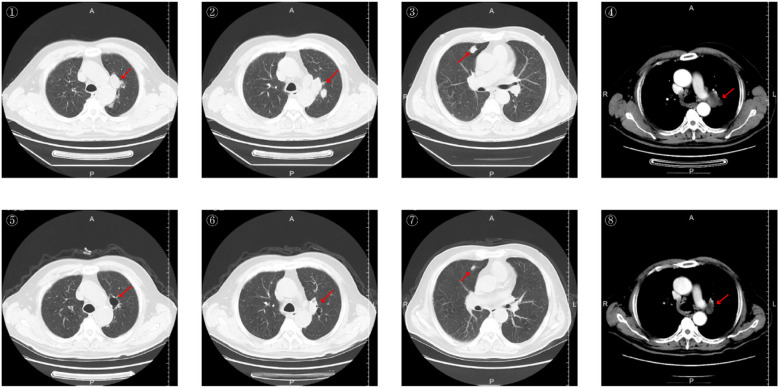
Chest CT images before and after treatment. Images ①②③④ show pre-treatment chest CT scans taken on July 17, 2025, revealing a mass at the hilum and within the left lung. Images ⑤⑥⑦⑧ display post-treatment chest CT scans taken on October 29, 2025, indicating tumor shrinkage compared to previous scans, with partial cavitation observed.
